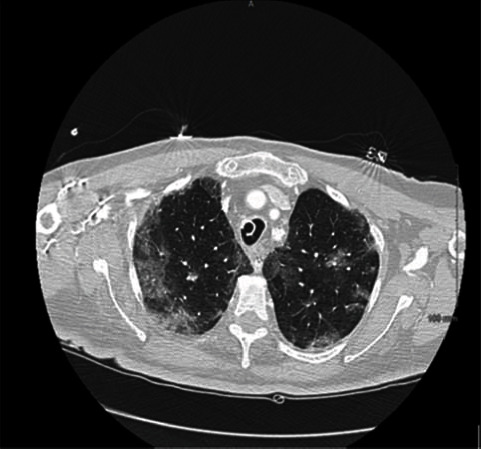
CT scan: bilateral ground glass opacities.
